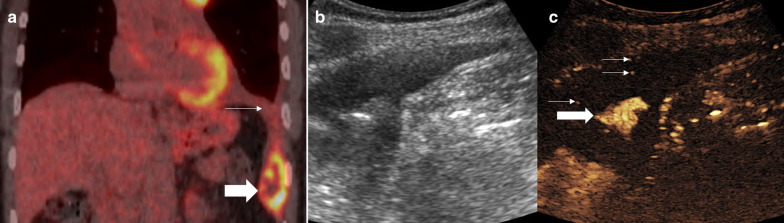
Patient with metastatic squamous cell carcinoma. a PET-CT examination shows a peripherally avid lesion in the costophrenic angle (arrow) with an associated pleural effusion (thin arrow). b, c Simultaneous B mode and CEUS images post-biopsy shows pooling of the UCA within the pleural effusion (thick arrow) which was static on dynamic imaging and single microbubbles (thin arrow) within the otherwise anechoic effusion. The findings are of post-biopsy haemorrhage which ceased without intervention
